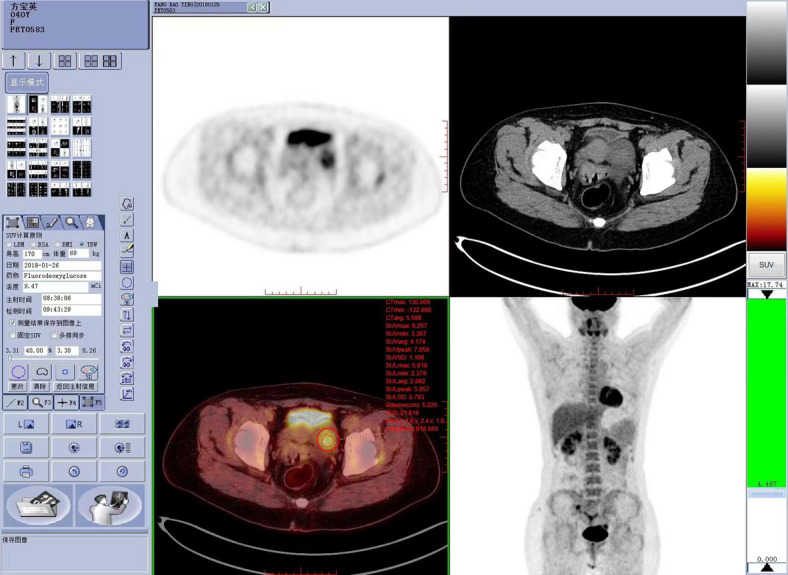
PET/CT working interface for intelligent diagnosis and treatment system (images and parameters).
